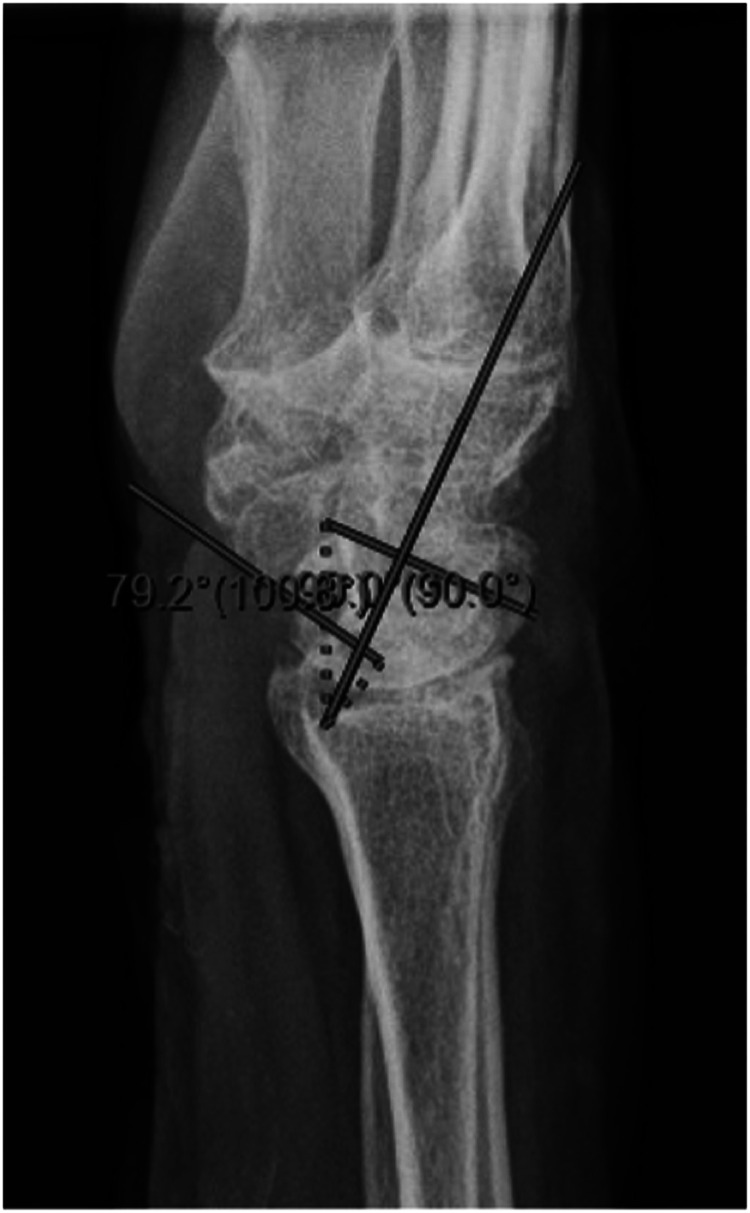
Preoperative right hand plain film lateral view x-ray.
